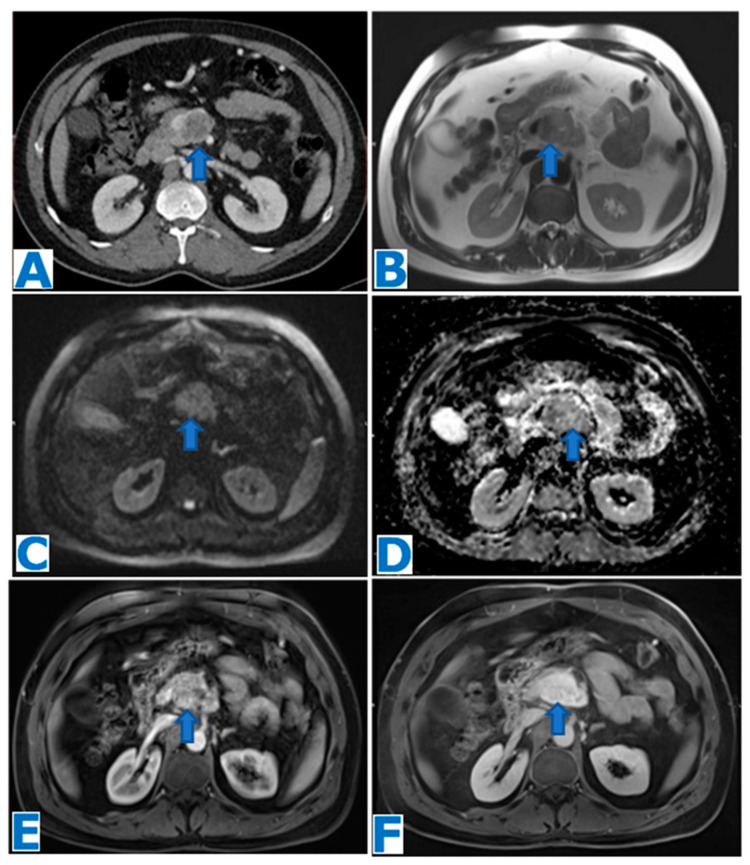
(A): axial CE portal venous phase CT, (B): axial T2WI, (C): DWI, (D): ADC, (E): axial CE pancreatic parenchymal phase FST1WI, (F): axial CE portal venous phase FST1WI. Primary pancreatic leiomyosarcoma incidentally discovered in a 53-year-old patient during a routine check-up. Note the heterogeneous mass within the pancreatic isthmus (arrow in (B)) with compression of the superior mesenteric and splenic vein (arrow in (A)). No biliary or pancreatic duct dilatation is observed. The lesion shows diffusion restriction (arrow in (C,D)) and hypervascularity, with progressive enhancement following intravenous contrast administration (arrow in (E,F)). EUS-guided FNB concluded PPLM. The patient underwent radiotherapy before surgery but then refused to be operated upon and developed hepatic and muscular metastases (not shown here). Stable disease was achieved with chemotherapy for five years, but it is currently progressing.
